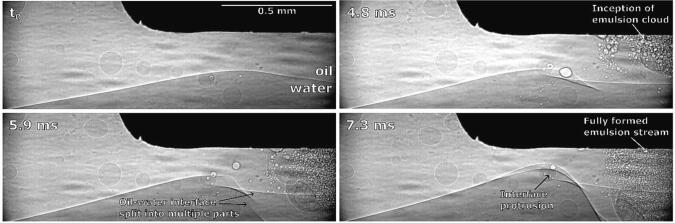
Synchrotron observations of interface indentation and interface peak formation below ultrasonic horn due to growing emulsion cloud.
